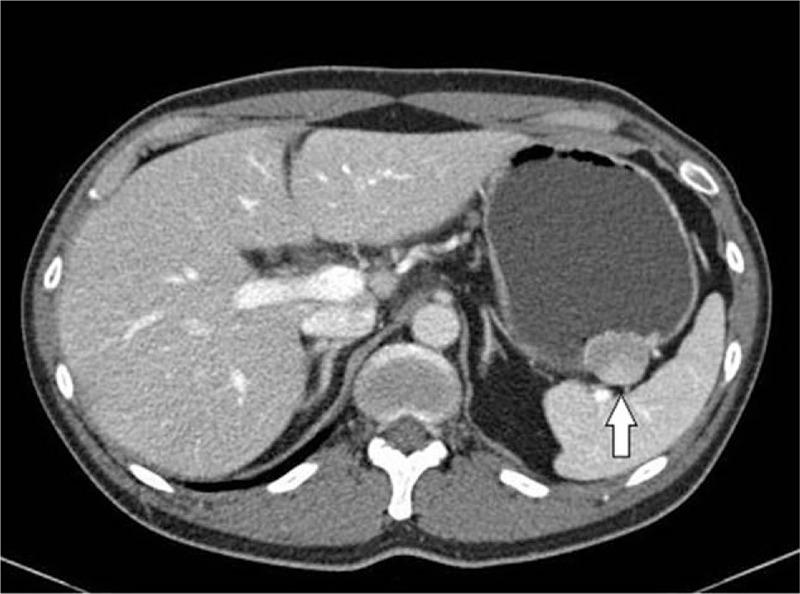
Abdominal computed tomography: a well-defined heterogeneously enhancing wall mass at the upper gastric body.
